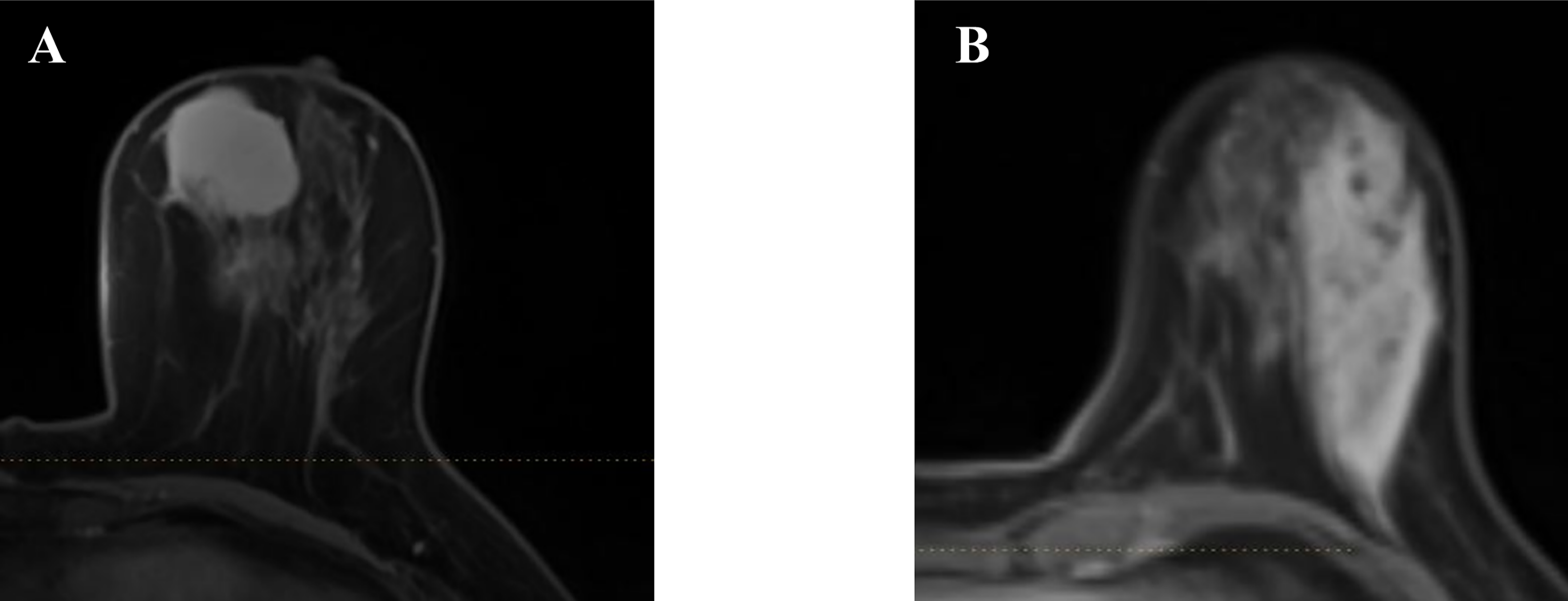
Two examples of the axial plane of contrast-enhanced T1-weighted MR images. (A) Malignant breast lesions. (B) Benign breast lesions.
